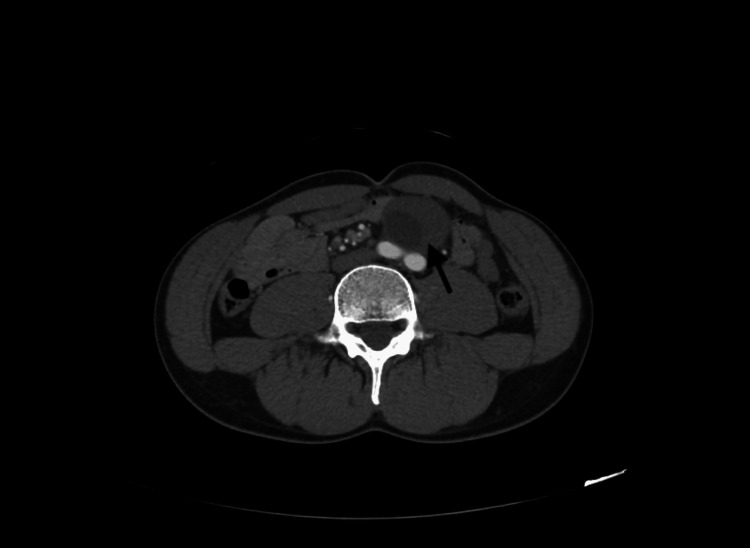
CT scan: axial view of para-aortic mass measuring 6.4 × 8.3 × 8.8 adjacent to the aortic bifurcation
